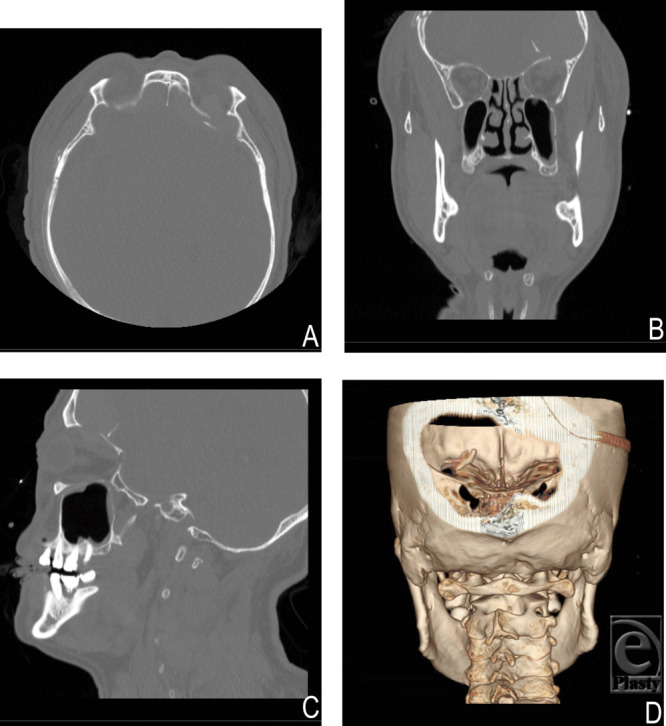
Facial computed tomography before the second operation: (*a*) axial view; (*b*) coronal view; (*c*) sagittal view; and (*d*) 3-dimensional reconstructive view. A displaced orbital roof fracture segment is seen.
